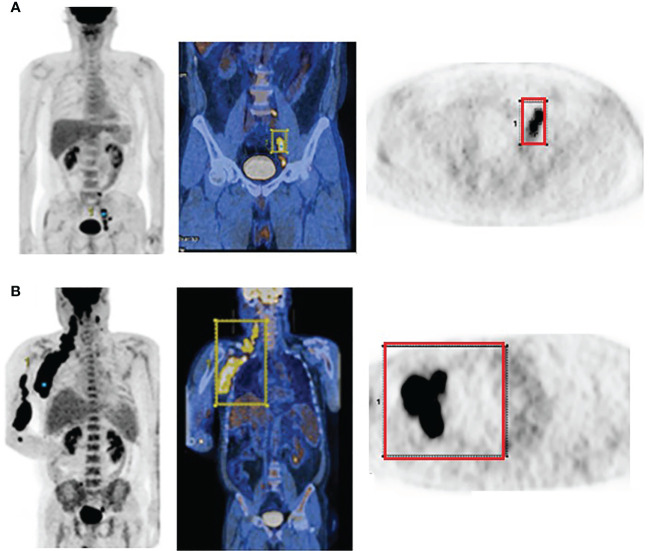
FDG PET and PET/CT maximum intensity projection images (left) and transaxial PET images (right) of representative DLBCL patients with a small (A) and large MBV (B). VOIs encompassing the lesions are also shown.
